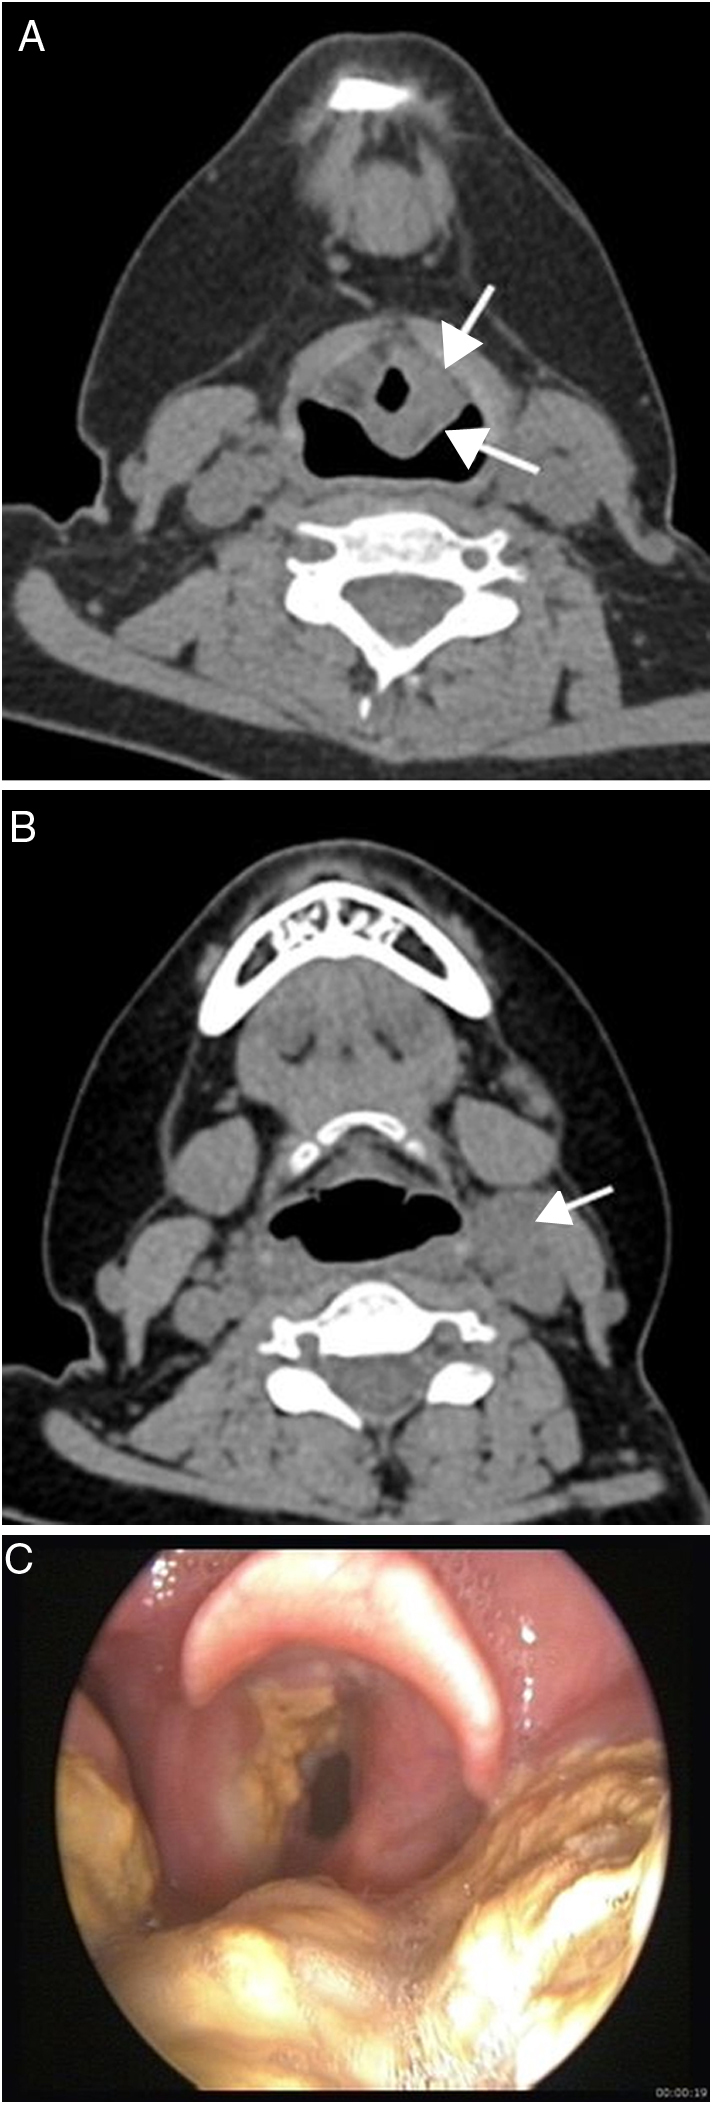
(A–C) 23 year-old woman with posttransplant lymphoproliferative disease, monomorphic, diffuse large B-cell type. Non-enhanced CT shows mass (arrows) (A) on left aryepiglottic fold and left cervical lymphadenopathy (arrow) (B). Endoscopic image (C) reveals irregular yellowish mass involving the left band ventricle, aryepiglottic fold and tongue base.
